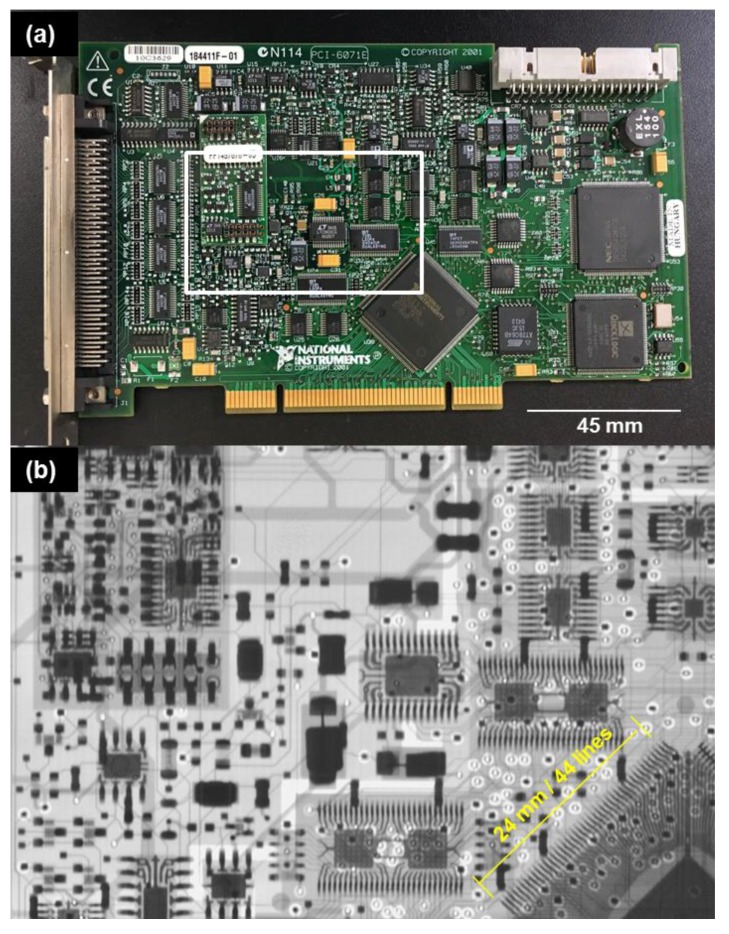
(a) Optical image and (b) X-ray image of printed circuit board taken at 45 kV/0.3 mA.
